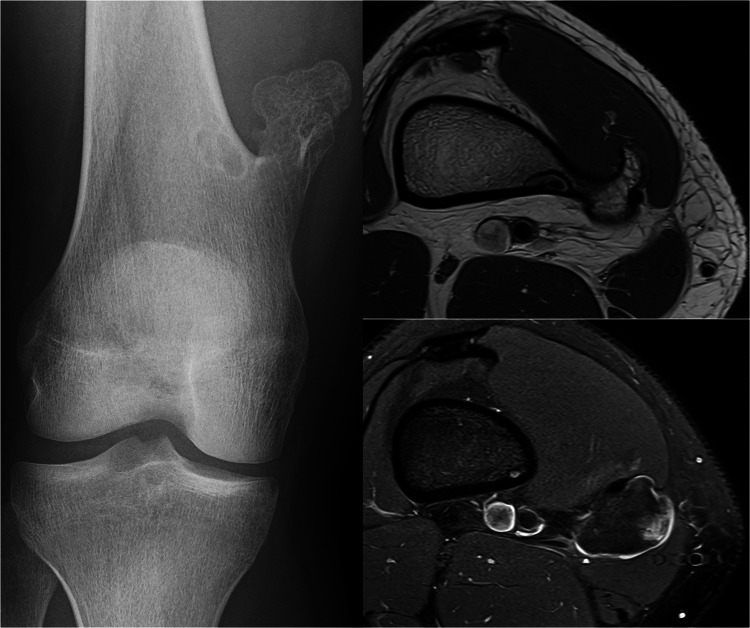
Eighteen-year-old male with pedunculated osteochondroma and non-ossifying fibroma. AP radiograph of the knee (left) shows a pedunculated osteochondroma arising from the medial distal femoral metaphysis, projecting away from the joint and demonstrating corticomedullary continuity. An eccentrically positioned NOF with central lucency and peripheral sclerosis is also present. Axial PD weighted image (top right) shows the pedunculated osteochondroma with corticomedullary continuity and moulding around the distal adductor magnus tendon. The NOF is seen in the posteromedial femoral cortex. Axial PDFS image (bottom right) confirms a slender, hyperintense chondral cap
